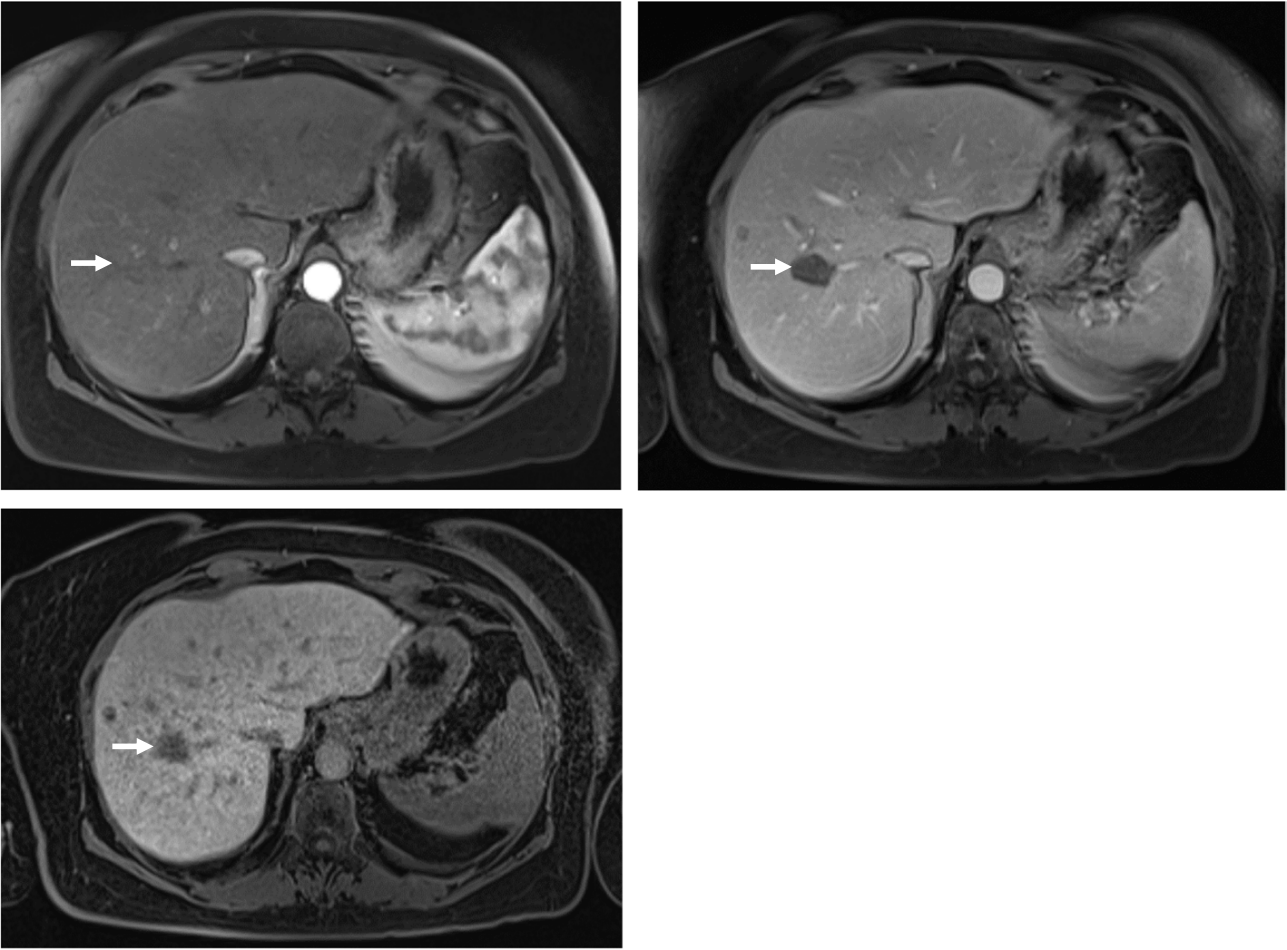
A 64-year-old female with non-alcoholic steatohepatitis. Axial T1-weighted post-gadolinium fat-saturated images demonstrate a segment 8, 25 mm lesion with no arterial phase hyperenhancement (APHE) (A), but with washout on portal venous phase (B) and hypointensity on hepatobiliary phase (C). Despite of the washout, the lesion would not be in keeping with HCC as per EASL, APASL and KLCA due to lack of APHE. As per LI-RADS, however, the lesion can be categorized as LI-RADS 4
